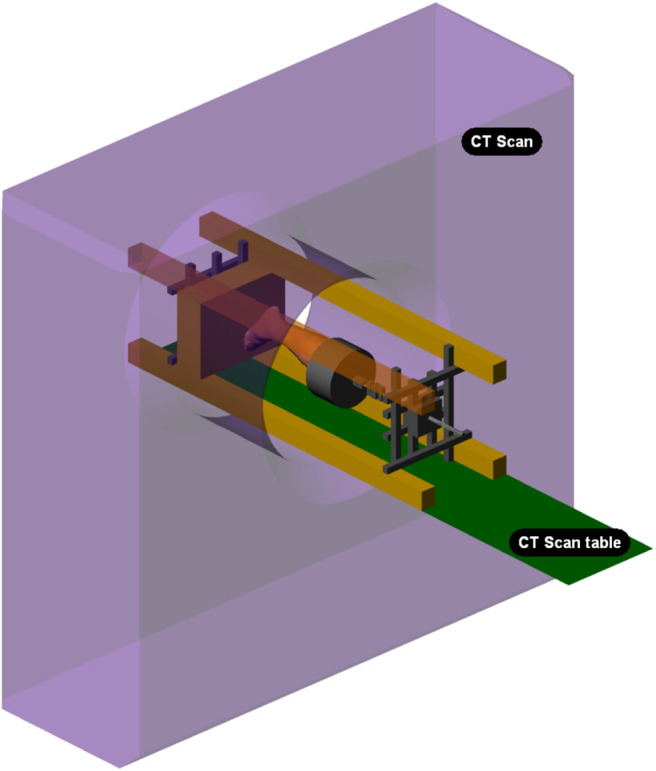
Test bench positioned in the CT scan.
